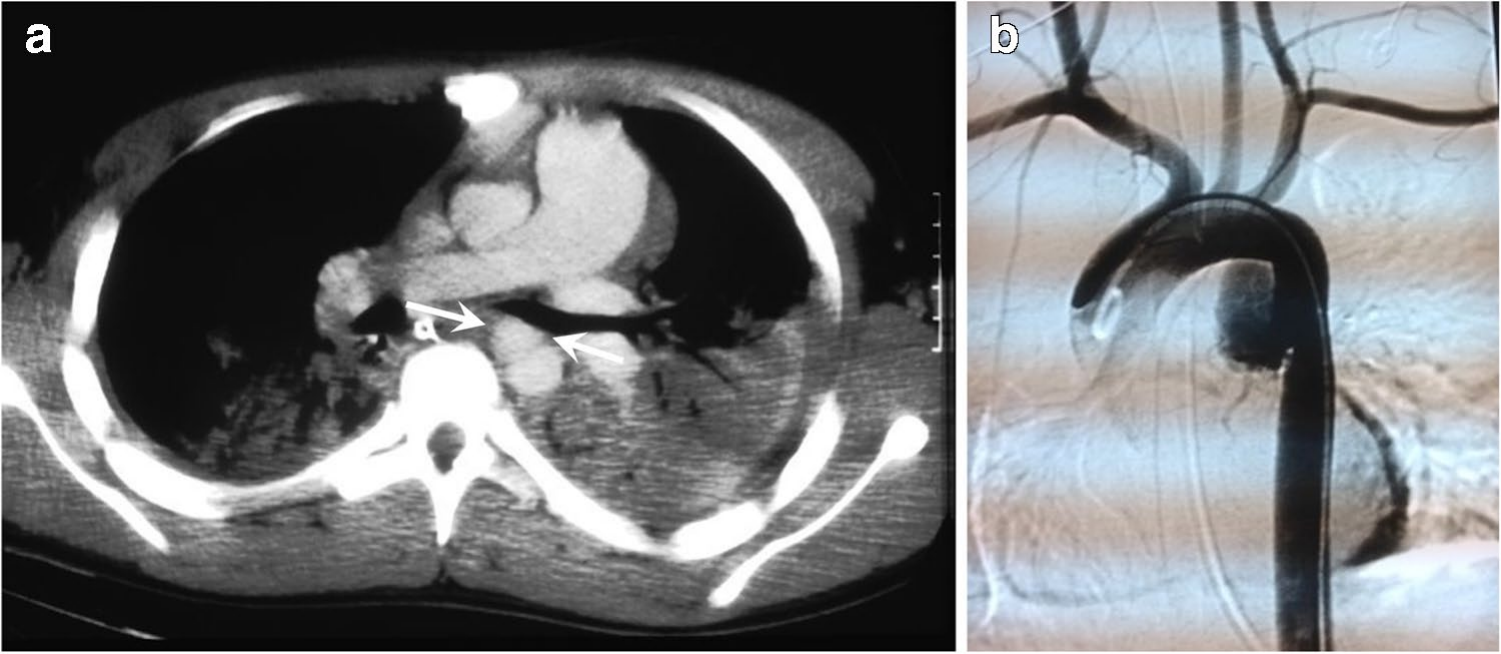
An 8-year-old boy with aortic pseudoaneurysm. a Contrast-enhanced computed tomography of the chest demonstrates a pseudoaneurysm in the proximal descending aorta (*arrows*). Bilateral consolidation/atelectasis is also noted in both lungs posteriorly. b Lateral view of digital subtraction aortic angiography demonstrates a traumatic pseudoaneurysm in the proximal descending aorta. Cases courtesy of Dr. David Manson from The Hospital for Sick Children, Toronto, Canada
